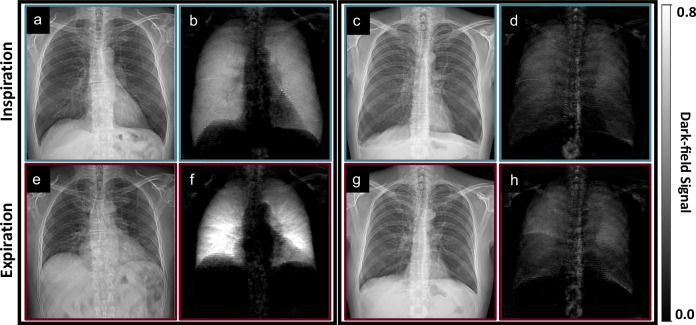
Attenuation (a, c, e, g) and dark-field (b, d, f, h) radiographs in inspiration (upper row) and expiration (lower row) in a 71-year-old healthy participant (lung volume in inspiration: 8.9 L; left array) and a 55-year old participant with advanced destructive emphysema (lung volume in inspiration: 10.6 L; right array). The emphysema patient exhibits typical signs of hyperinflation in the conventional radiograph and a lower dark-field signal in the dark-field radiograph when compared to the healthy participant. While the lung area decreases from inspiration to expiration images, the dark-field signal intensity increases. Both effects appear to be weaker for the emphysema patient. The same window and level settings were applied within the respective modality for all participants
